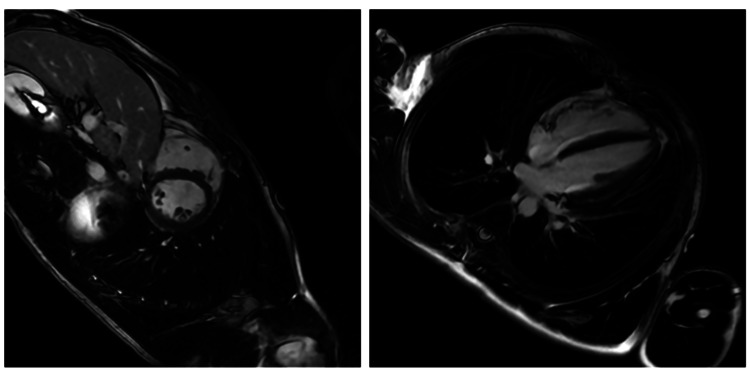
Cardiac MRI Left: Short-axis view at mid-ventricular level shows no abnormal late gadolinium enhancement in the myocardium. Right: Long-axis 4-chamber view shows no abnormal late gadolinium enhancement. (Images were obtained following injection of 26mL intravenous (IV) contrast and after T1 suppression).
